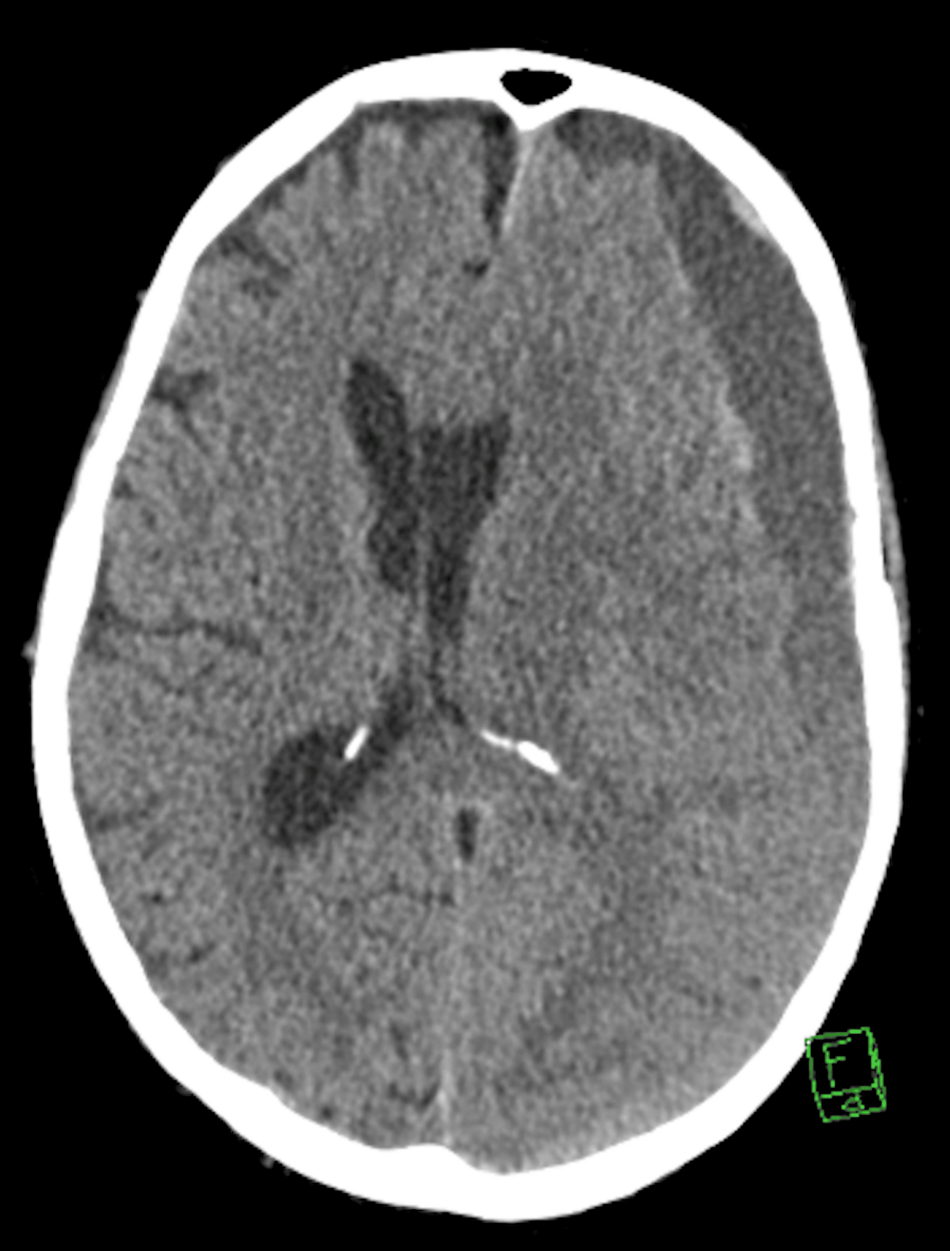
Non-contrast head CT showing a large left-sided subdural hematoma with mixed densities suggesting an acute-on-chronic hemorrhage. The hematoma measured 2.0 cm in greatest depth with 1.0 cm of rightward midline shift.
